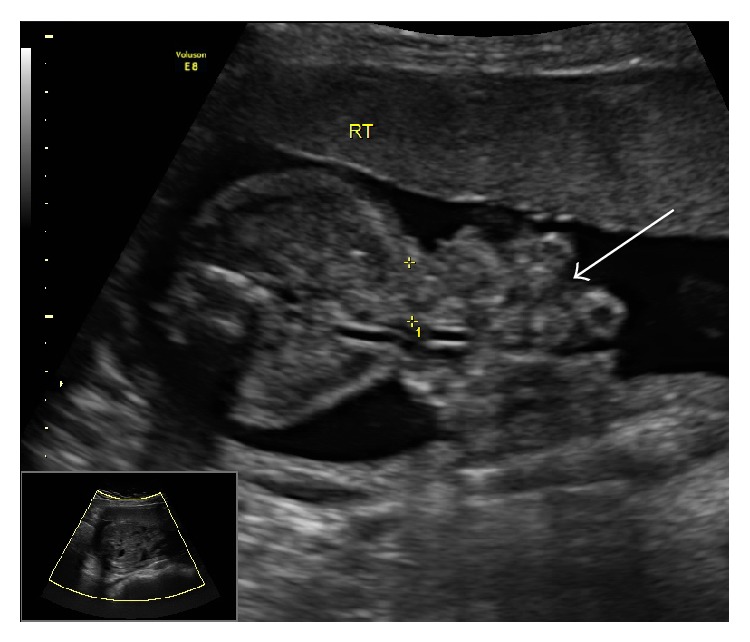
Ultrasound image showing small anterior wall defect beside umbilical cord insertion with small bowel herniation.
